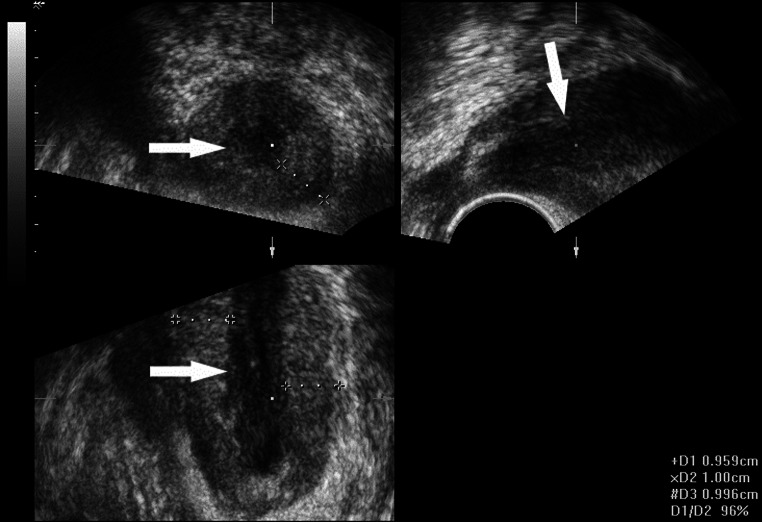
Multiplanar reconstruction: axial, sagittal, and coronal views of anal canal show hypertrophy of internal anal sphincter (10 mm thickness; calipers) and hypoechoic mucosa (*arrows*) devoid of normal folded patternTomographic US imaging, like CT or MRI scans, enables visualization of the whole anal canal or just the lesion in consecutive planes (e.g., axial) to optimally present the whole pathology in one display (Fig. 2b);Volume render mode enables the assessment of the content of 3D presentation of the anal canal and surrounding tissues more precisely [11], using different post-processing techniques:Minimum intensity projection, which employs an algorithm displaying an interactive (e.g., with 360° rotation around the longitudinal axis of the anal canal) 3D image of dark (hypoechoic or anechoic) structures, e.g., hypoechoic internal anal sphincter or hypoechoic scar.Maximum intensity projection, which employs an algorithm displaying an interactive (e.g., with 360° rotation around the longitudinal axis of the anal canal) 3D image of bright (hyperechoic) structures, e.g., hyperechoic contents of a distended rectal ampulla in a patient with anal canal stenosis (Fig. 1b). Such images may resemble radiologic proctography with uropolinum.Static volume contrast imaging, which is a thin slice volume rendering technique with combination of various algorithms, including surface algorithm (Fig. 2b). It renders slices as thick as 2–10 mm and provides higher contrast and less noise than a conventional display.
